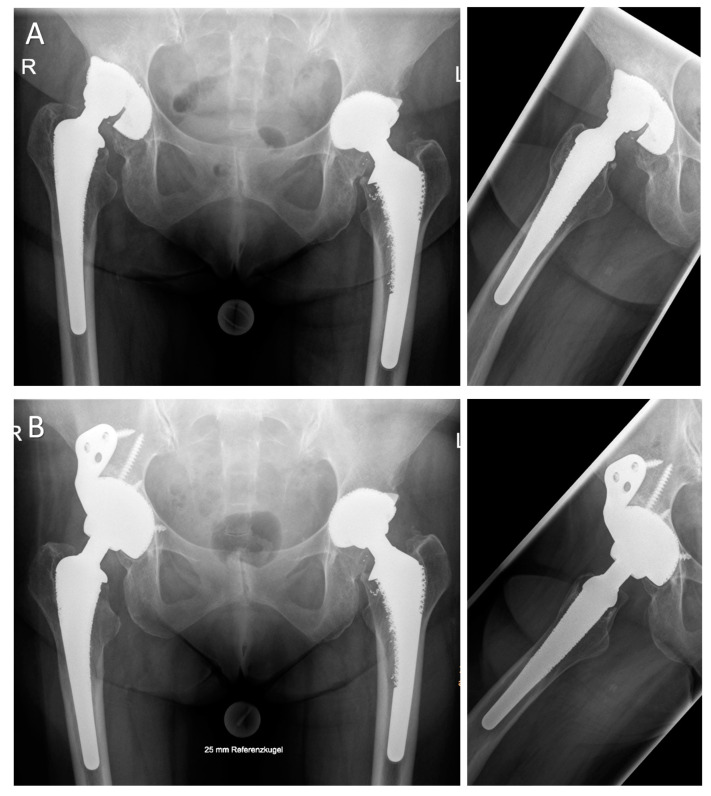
(A) A 63-year-old female patient with failure of the acetabular implant and subsequent cranial migration 18 years after the index procedure (a.p. and axial views). Intraoperative defect evaluation revealed a large uncontained cranio-lateral defect (“up-and-out”) with rim destruction of more than 30% (Paprosky type IIIA defect). (B) Radiographs 24 months postoperatively revealed a stable acetabular implant augmented with multiple screws through the implant’s body and the iliac flange (HHS: 25 preoperatively, 81 postoperatively; OT 79 min).
